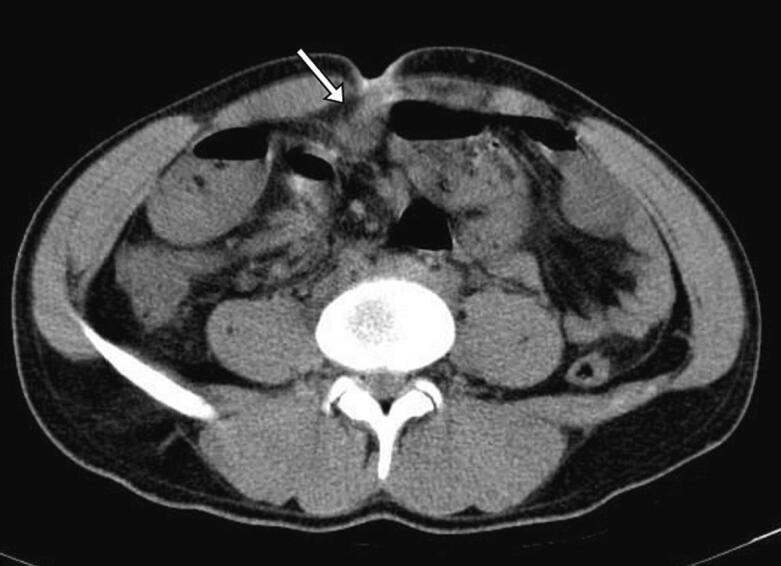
Abdominopelvic CT scan, Axial View, an arrow points to an adhesion band connecting the umbilicus to the intestinal wall, later identified as the fibrous band of the OMD.
